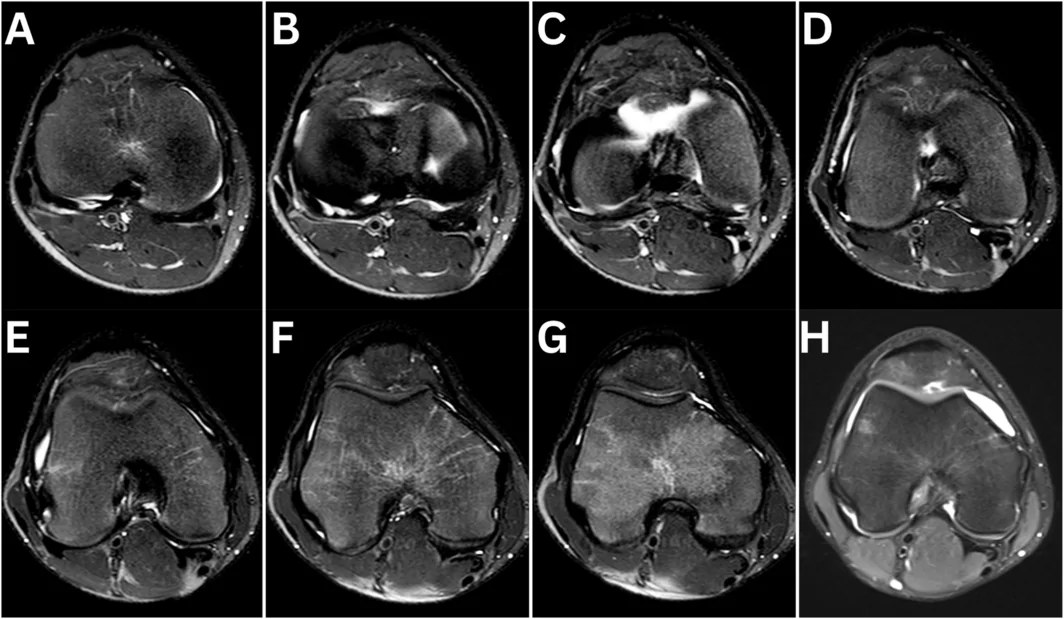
Follow‐up axial MRI at 12 months. T2‐weighted fat‐suppressed images (A–H) demonstrate restoration of ACL continuity, with a continuous, taut, low‐signal ligament band of normal oblique orientation within the intercondylar notch (D–G). The prior pivot‐shift bone‐marrow edema has resolved, with only trace physiologic joint fluid and no secondary signs of ACL deficiency.
